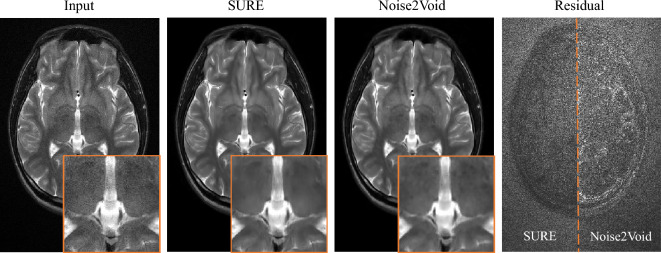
Results of denoising real MR images without ground-truth data. Our proposed SURE-based approach demonstrates superior performance compared to the reference method Noise2Void in terms of image sharpness and clarity. The residual images show the difference between the input and the denoised images, i.e., the removed noise.
